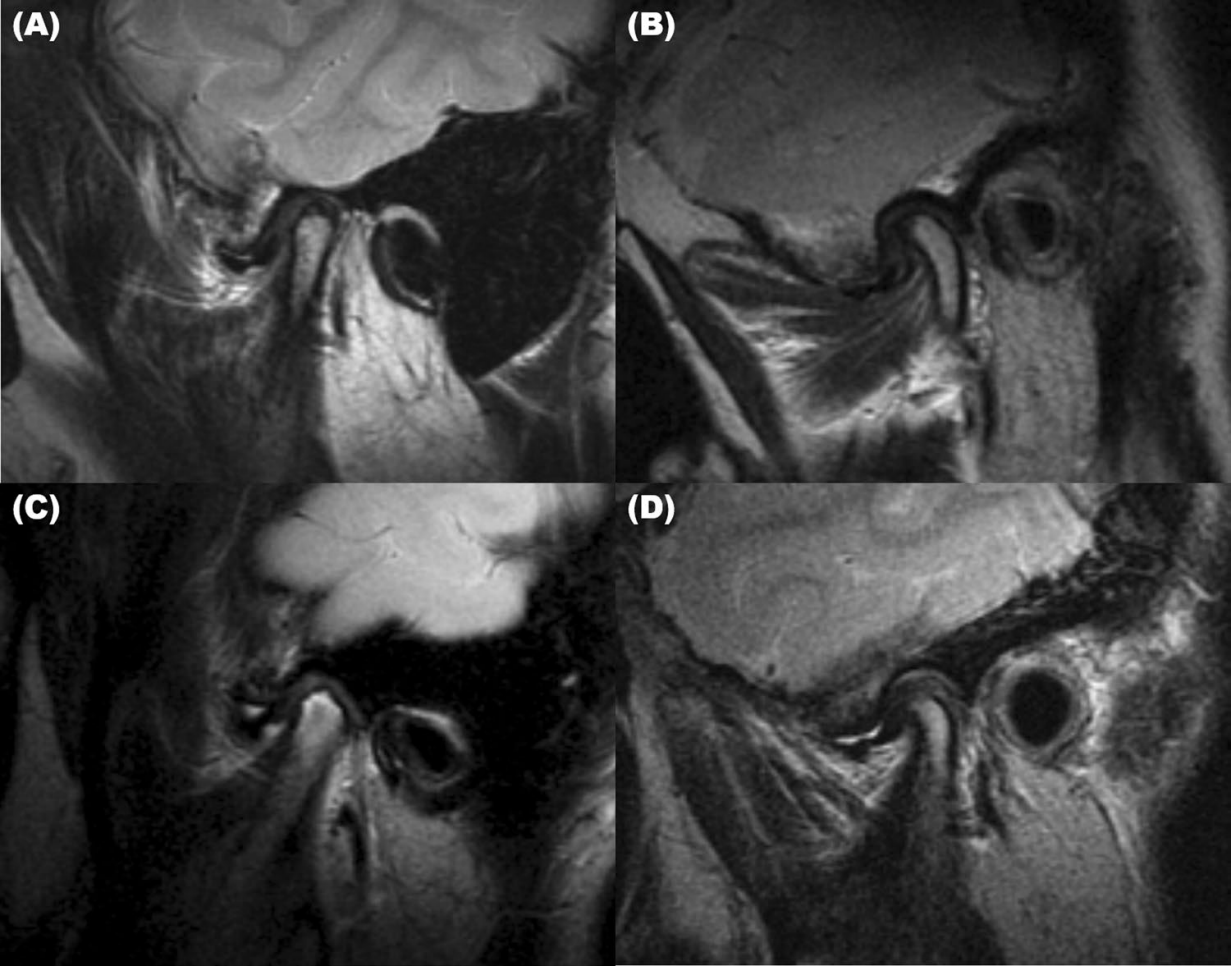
Magnetic resonance imaging (MRI) features of the abnormal temporomandibular joint disc and mandibular condyle. (A) Anterior disc displacement. Sagittal oblique T2-weighted image (T2WI) acquired in the closed-mouth position shows an anteriorly displaced disc. (B) Disc deformity. Sagittal T2WI shows a dysmorphic and anteriorly displaced disc. (C) Condylar degeneration. T2WI shows arthritis of the mandibular condyle. (D) Effusion. T2WI shows clearly delineated articular fluid collection with hyperintensity, degeneration of the mandibular condyle, and an anteriorly displaced disc.
